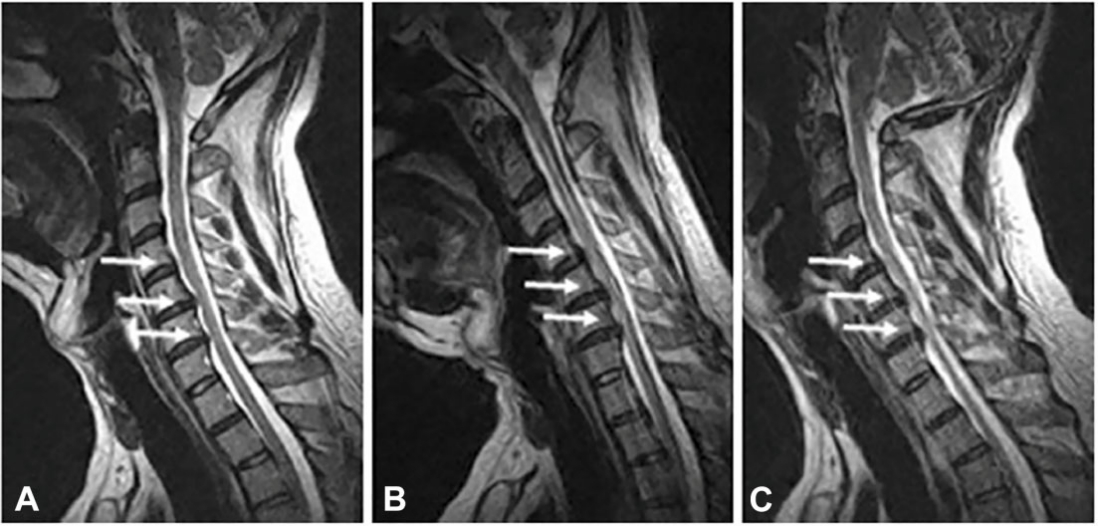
Dynamic cervical spinal cord compression on MRI. A neutral (A) and flexion (B) and extension (C) T2 MRI showing the effect of movement on spinal cord compression. Here flexion of the spine unmasks spinal cord compression not clear in neutral imaging. Taken from—Lao et al.^
